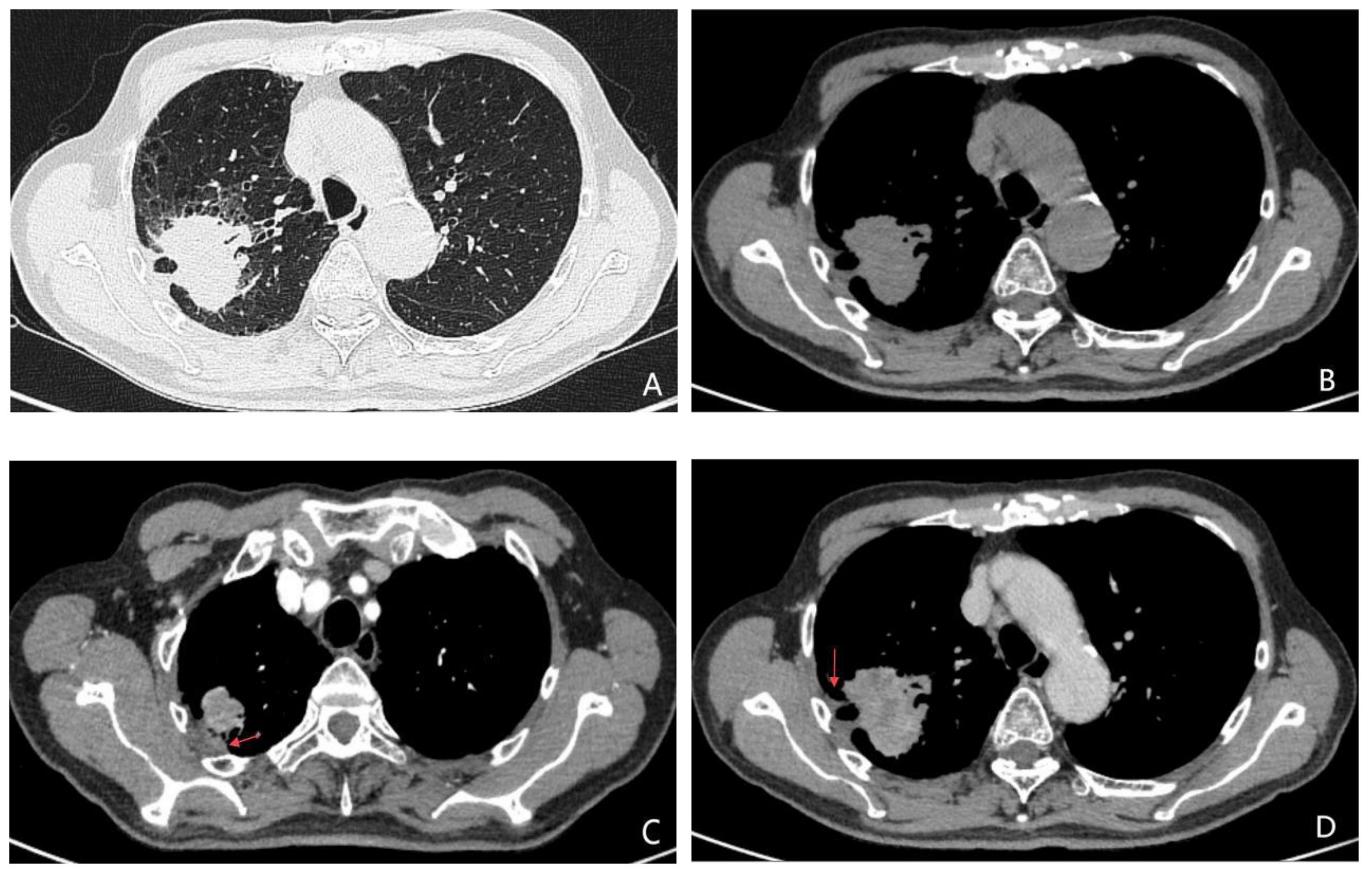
(A-D) A 74-year-old male patient with lung adenocarcinoma, the genetic testing result was EGFR 21L858R mutation (+). Unenhanced CT images (A, B) demonstrate an irregular soft-tissue nodule with indistinct margins and adjacent pleural thickening in the right upper lobe. Contrast-enhanced CT during arterial (C) and venous (D) phases reveals heterogeneous marked enhancement of the lesion, with no significant lymphadenopathy in the mediastinum.
